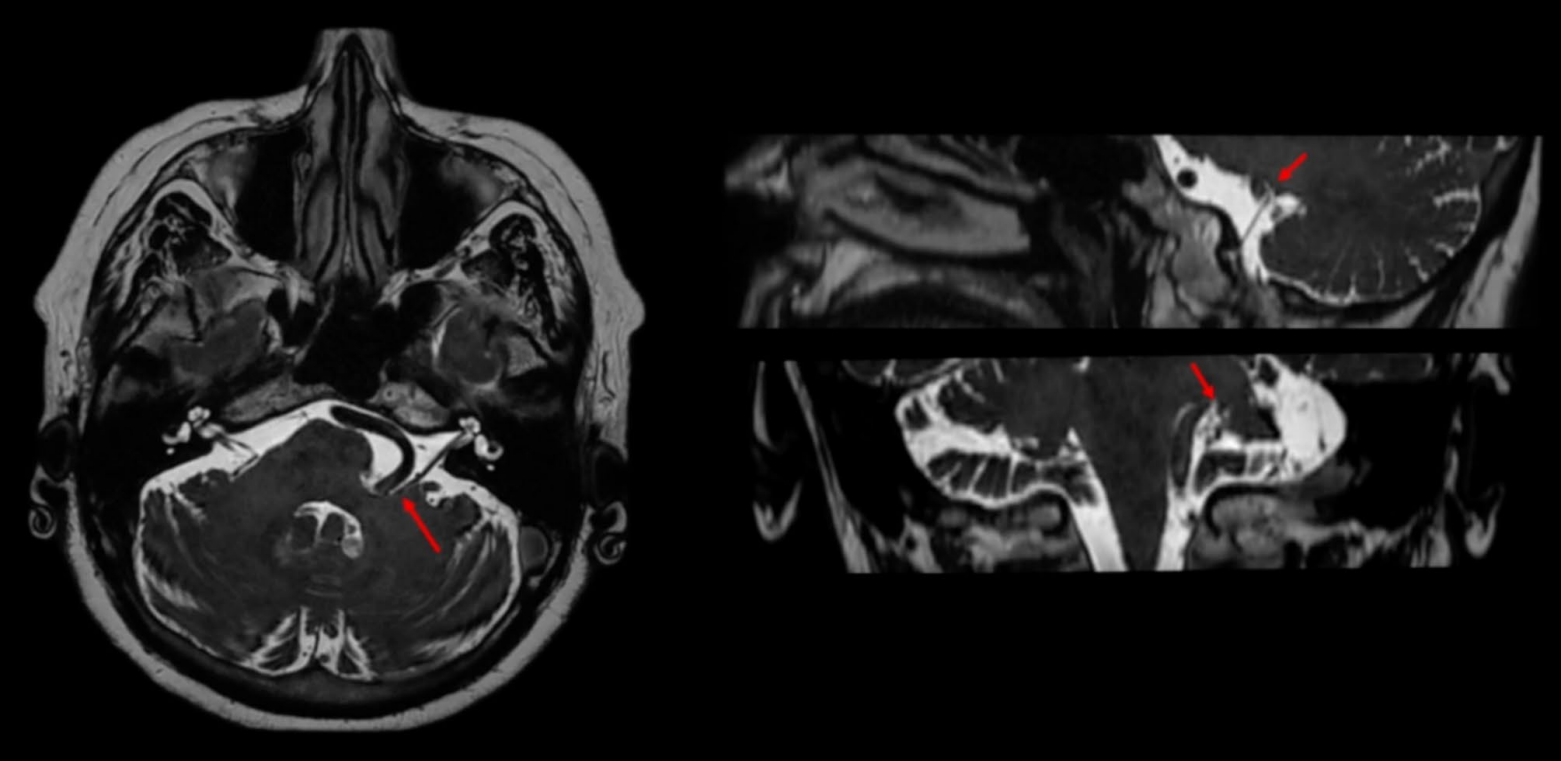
The brain MRI with FIESTA sequences in three planes showed a potential neurovascular conflict between the left vertebral artery and the facial nerve along its proximal tract near the root exit zone pointed in with the red arrow. The vertebral artery was aberrant in his course with a loop occupying the left cerebellopontine angle
